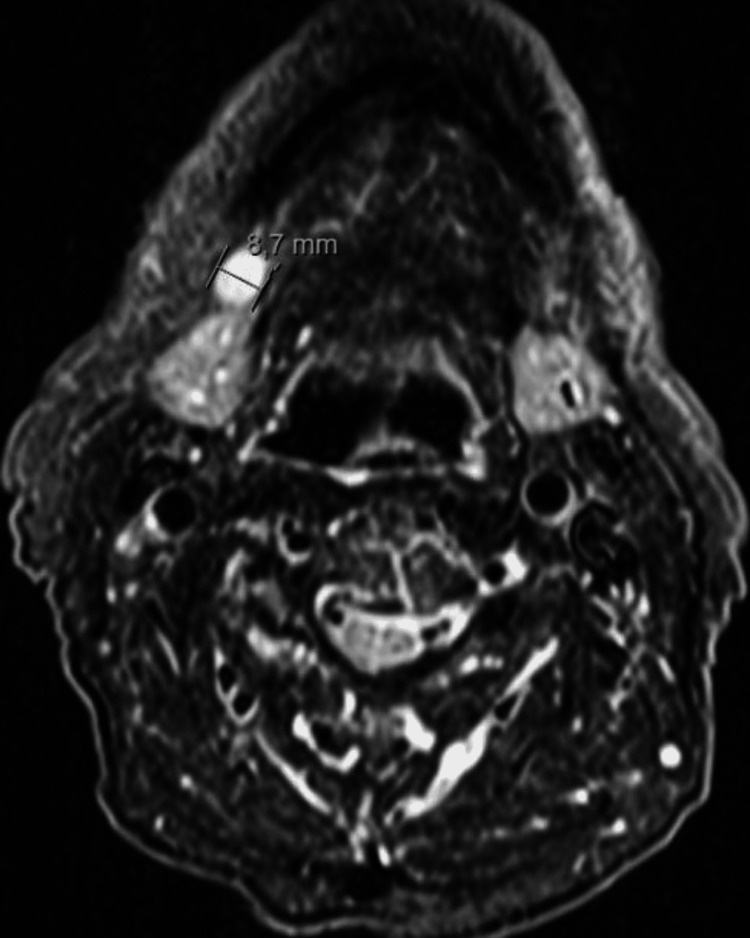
MRI scan highlighting the largest right level I lymph node
